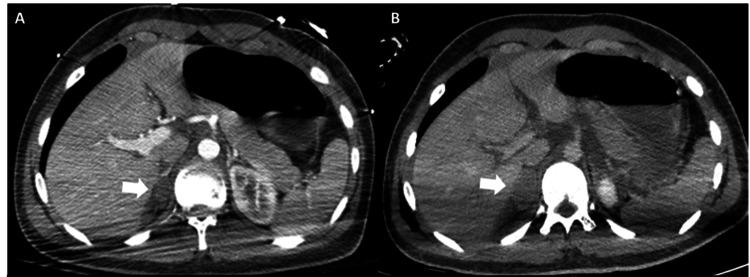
Abdominal CT findings A. Abdominal CT scan on admission: the arrow indicates a hypodense lesion located in the right adrenal gland, suggesting hematoma (2.48 x 1.61 cm).b. B. The CT scan shows that the right adrenal gland hematoma dimension increased nine hours after admission (4 x 2.7 cm). Diffuse perihepatic and peripancreatic hemorrhagic fluid collection can also be seen.
